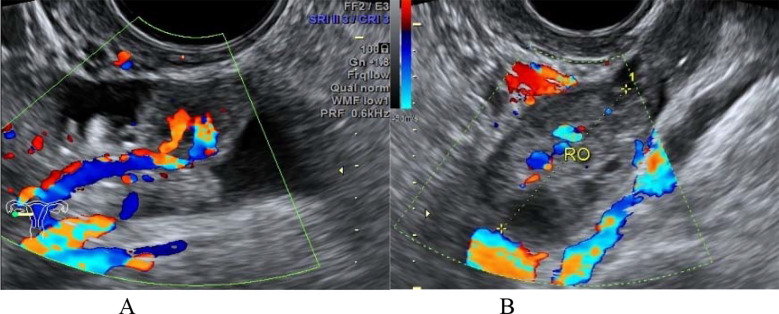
A patient with bilateral type I PFTC (No. 3). (A): the transvaginal color Doppler ultrasound showed the sausage-shaped cystic-solid mass in right paraovarian region; (B): the right normal ovary.
